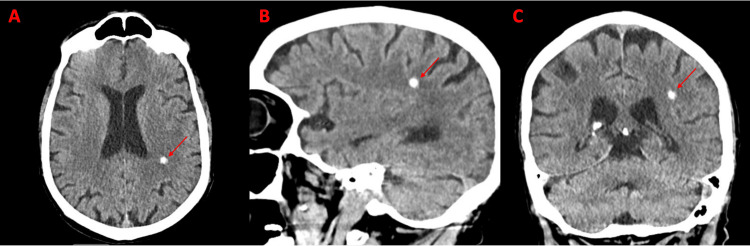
CT Head without contrast findings suggest a small focus of intraparenchymal hemorrhage involving the left frontoparietal centrum semiovale (Shown by red arrows). A: Axial View, B: Sagittal View, C: Coronal View.
